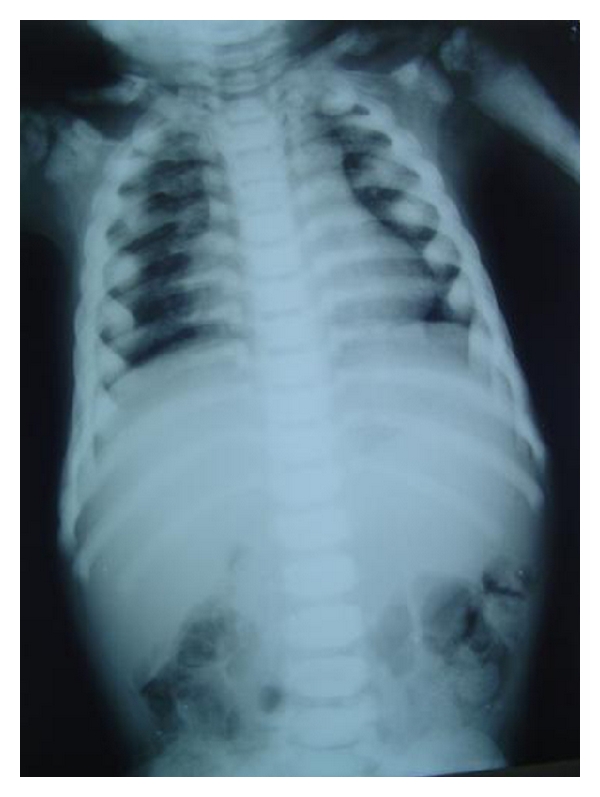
Chest radiograph showing long and narrow thorax (barrel-shaped chest) with anterior compression.
